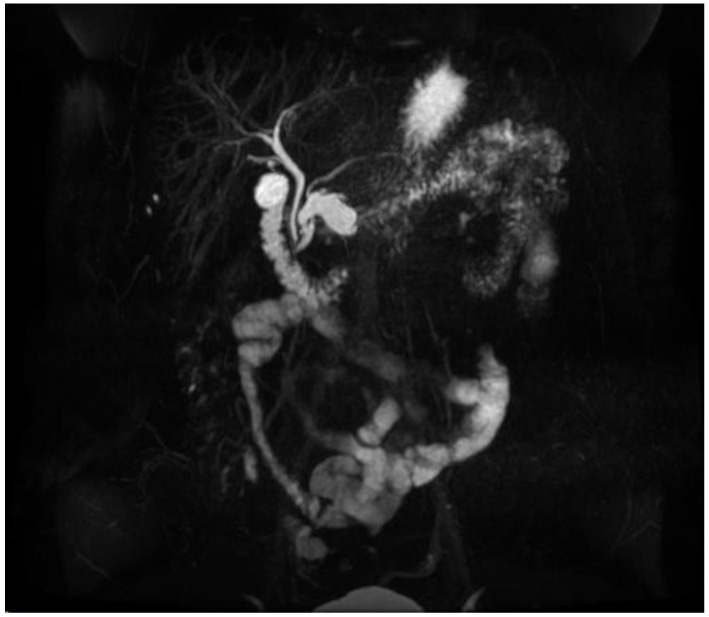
MRI image of mixed IPMN.
